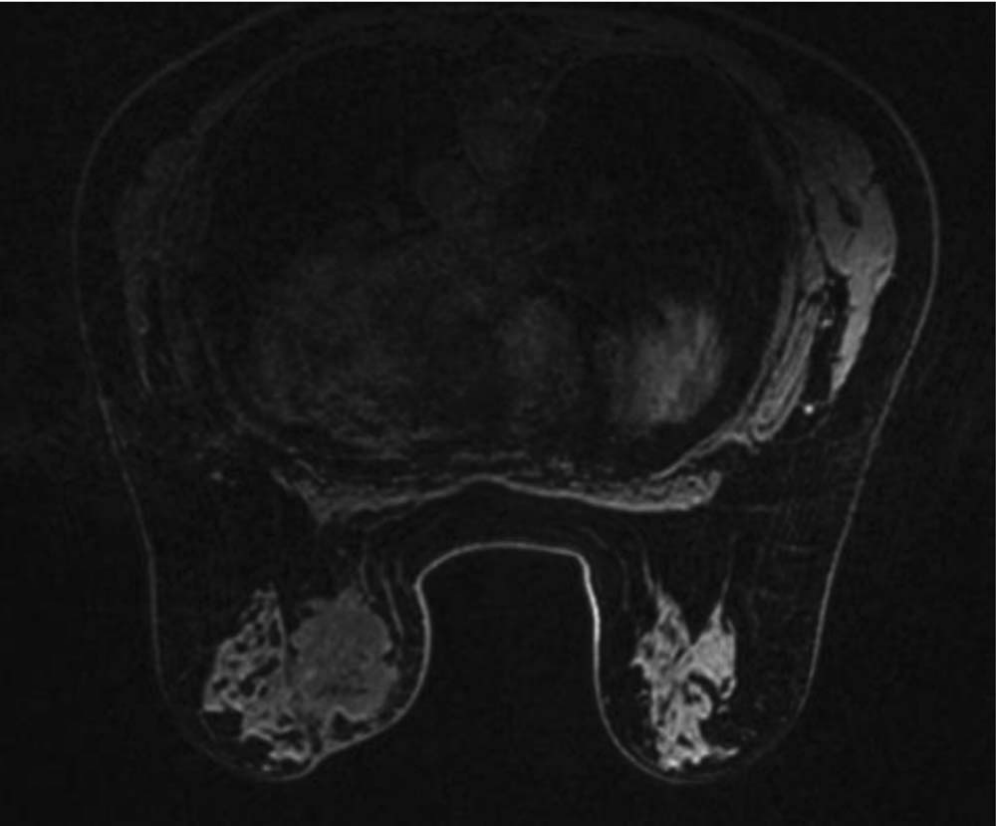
MRI. A 3.7 × 5.6 × 4.7 cm mass at the lower inner portion of the left breast. MRI = magnetic resonance imaging.
